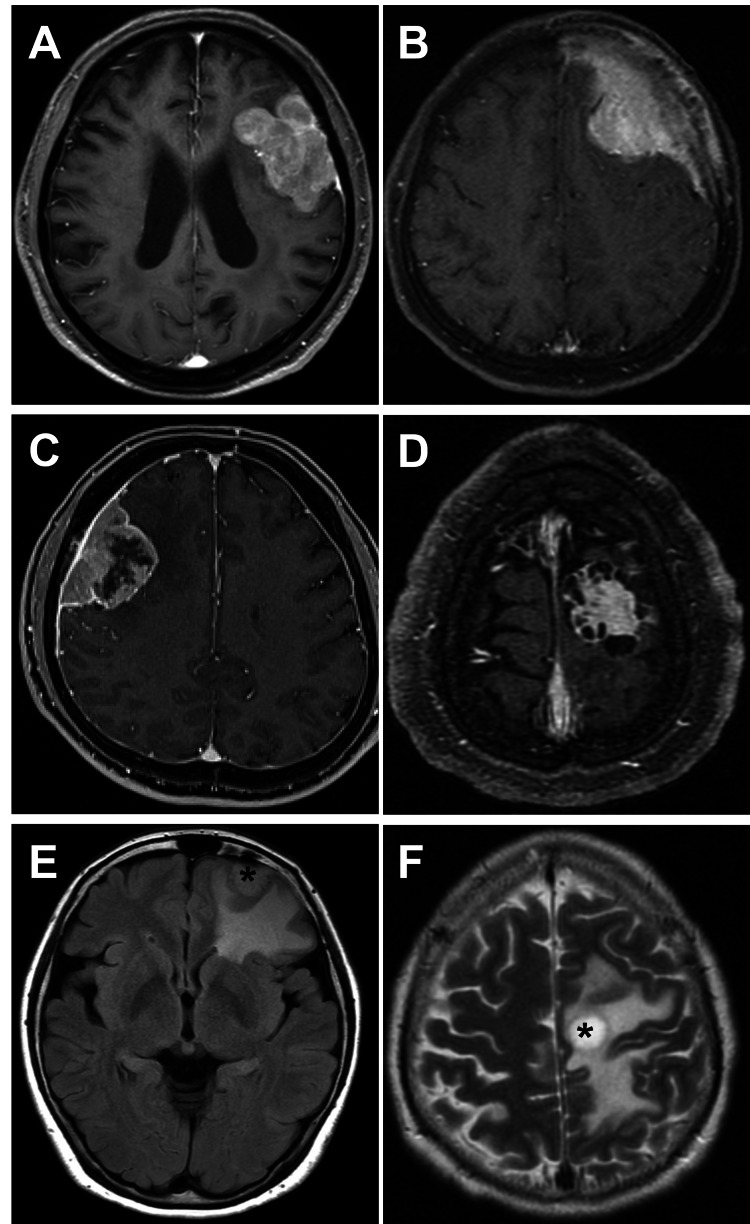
Representative MRI MRI showing representative axial images of irregular tumor shape, such as lobulated appearance (A) or mushrooming appearance (B), and heterogeneous tumor enhancement (C and D) on contrast-enhanced T1-weighted imaging and peritumoral brain edema on fluid-attenuated inversion recovery imaging (E) and T2-weighted imaging (F). The asterisk indicates tumor location MRI: magnetic resonance imaging
